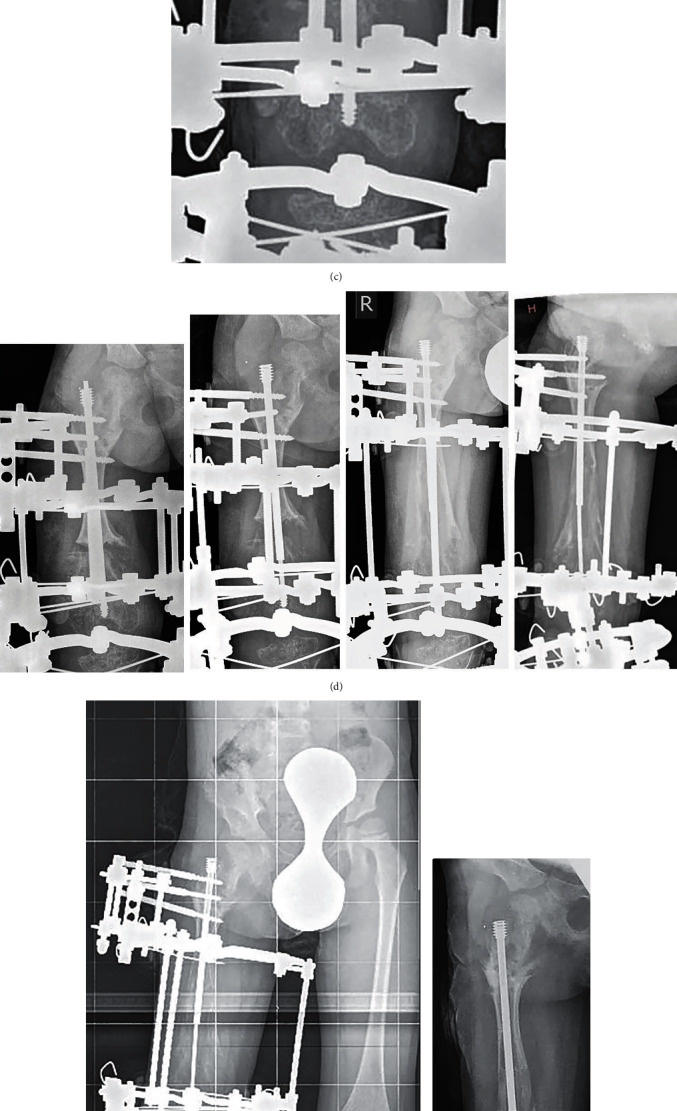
Radiographs of patient L.: (a) full-length standing radiograph and lateral view before surgery; (b) intraoperative radiograph performed after corrective osteotomy and insertion of the rod; (c) intraoperative radiograph done after frame application, by the end of surgery; (d) consecutive Antero-posterior (AP) radiographs demonstrating progressive lengthening of femur and bone union by the end of treatment period. Note excellent position of threaded ends of rods parts into distal epiphysis and apophysis of greater trochanter; (e) full-size standing radiograph of lower limb, satisfactory alignment of right limb; (f) position of the rod after frame removal: no rod migration neither bending. Threaded ends are into apophysis and distal epiphysis; (g) in three months after frame removal.
